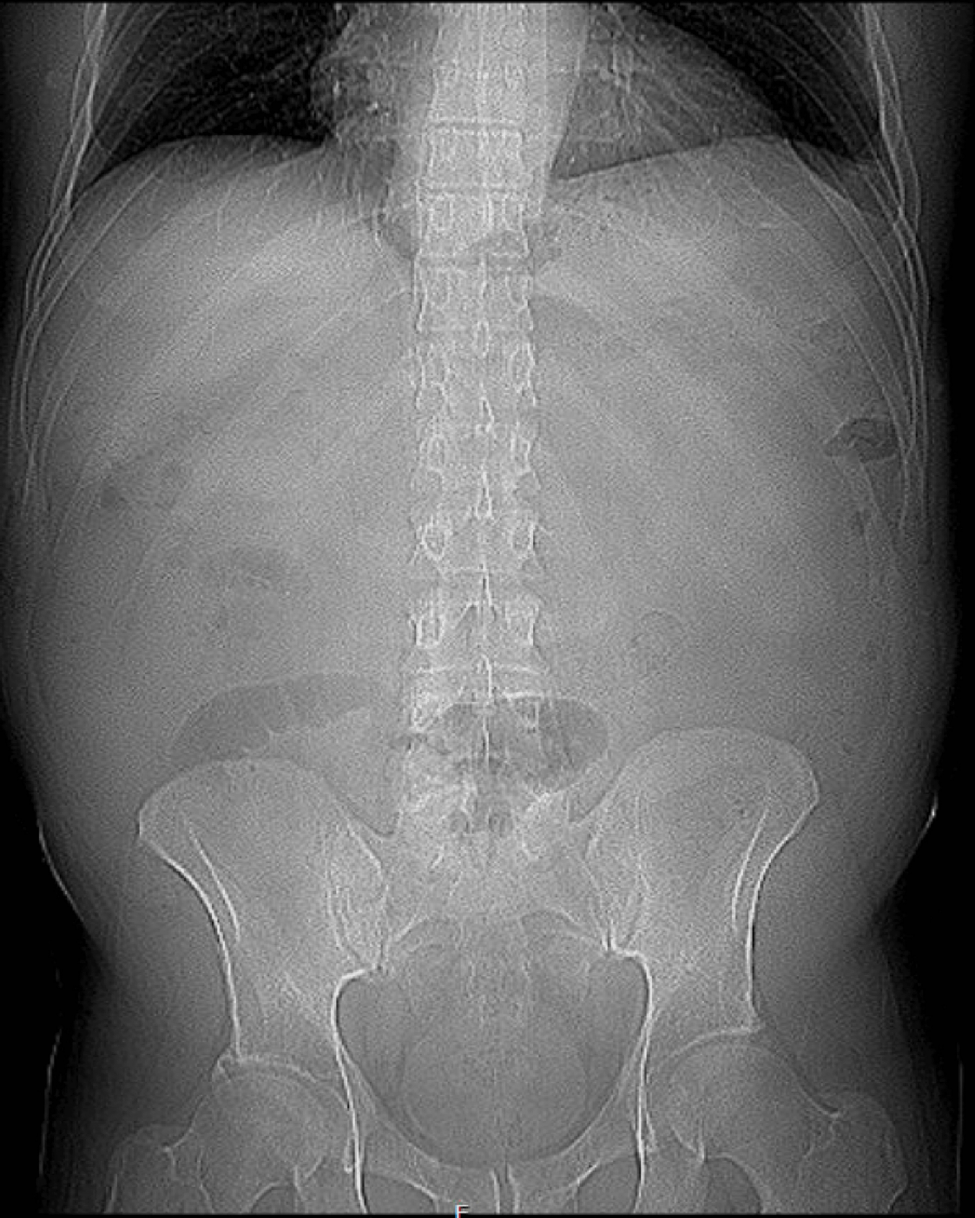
The abdominal X-ray demonstrates nonspecific bowel gas distribution without radiographic evidence of obstruction or perforation. No hernia sac is visualized, which is consistent with the known limitations of plain films in detecting Spigelian hernias. Further evaluation with contrast-enhanced CT is recommended for definitive diagnosis and assessment.
